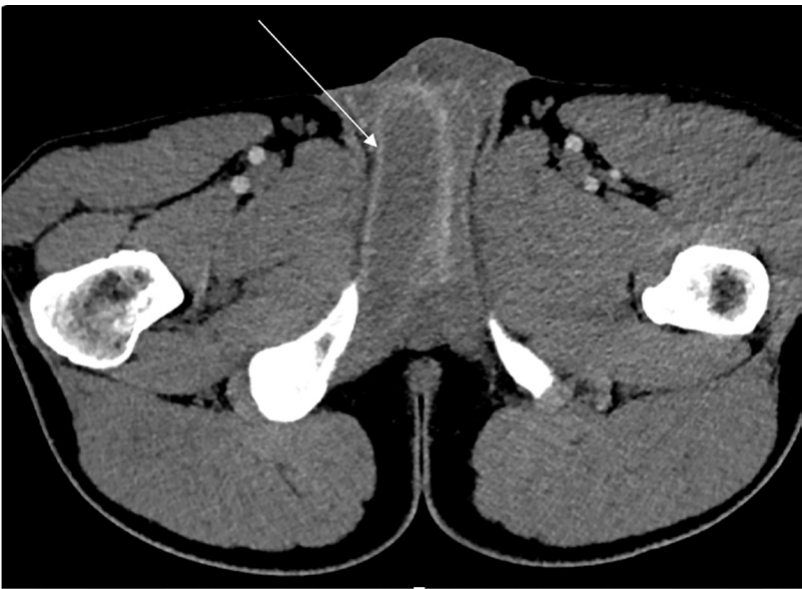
Non-contrasted computed tomography of the pelvis demonstrating asymmetry of the right and left corpus cavernosum.
